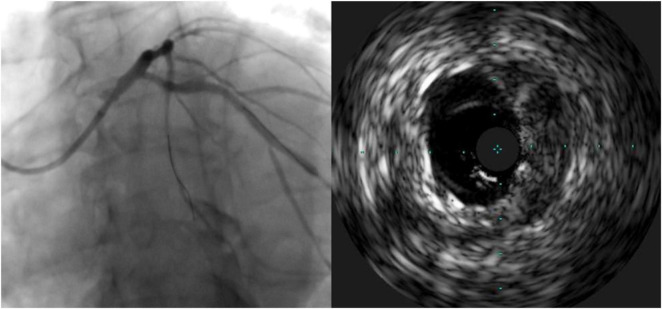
Case of geographic miss showing incomplete ostial coverage by angiography and intravascular ultrasound.
